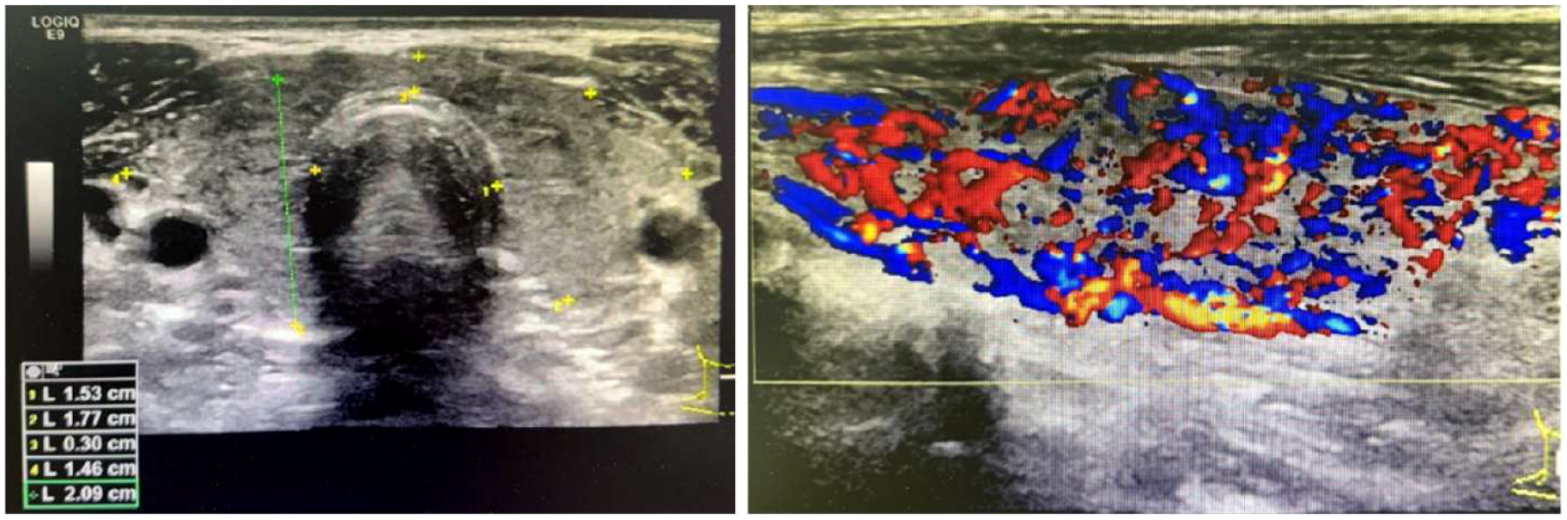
Thyroid ultrasonography. The thyroid gland was mildly enlarged with diffuse parenchymal echotexture abnormalities.
